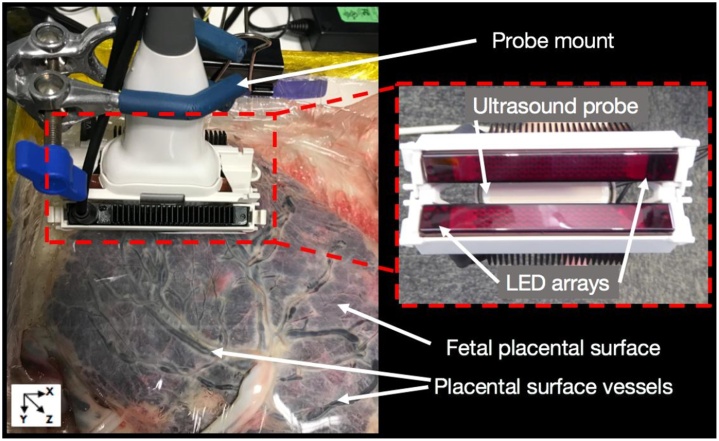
PAI system using light-emitting diode (LED) arrays mounted on the ultrasound probe for human placenta imaging [61].
